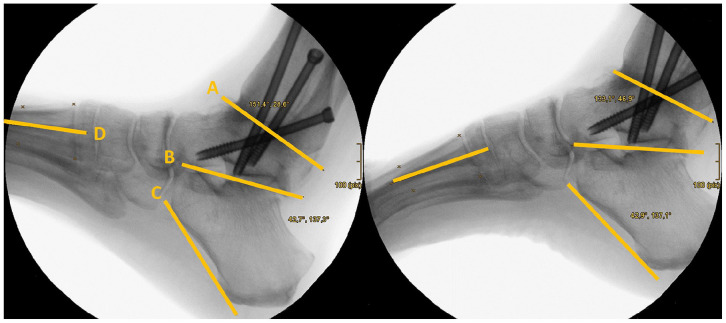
Lateral radiographs of the arthrodesed ankle in (a) maximum dorsiflexion and (b) maximum plantarflexion. Shown are the auxiliary lines marking bony landmarks of (A) tibia, (B) talus, (C) calcaneus, and (D) metatarsal 1, used for determining the range of motion (ROM) of the subtalar and medial tarsal joints. (complete ROM: difference of angles between A or B and D, subtalar ROM: difference of angles between A or B and C).
